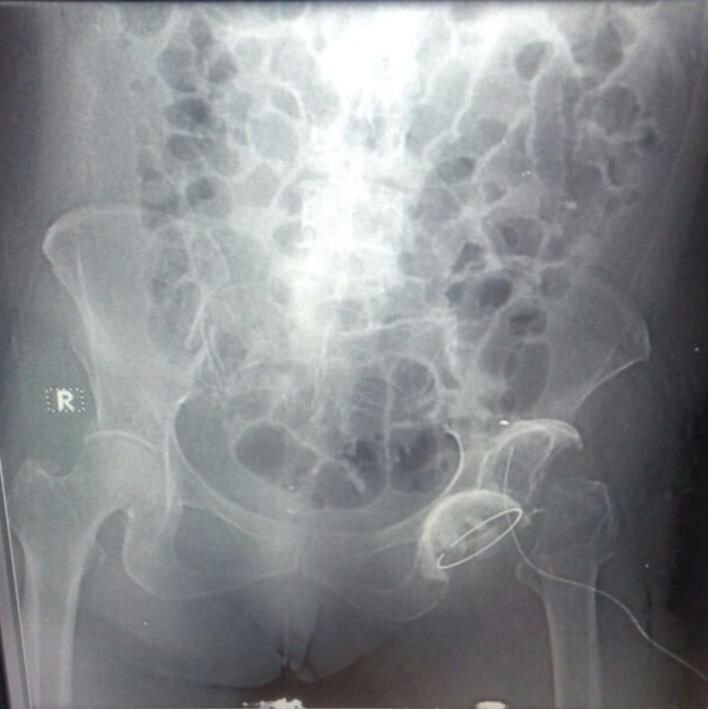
Plain radiograph after the first stage of surgery shows the application of a left acetabular cap and osteotomy of the left femoral neck.
